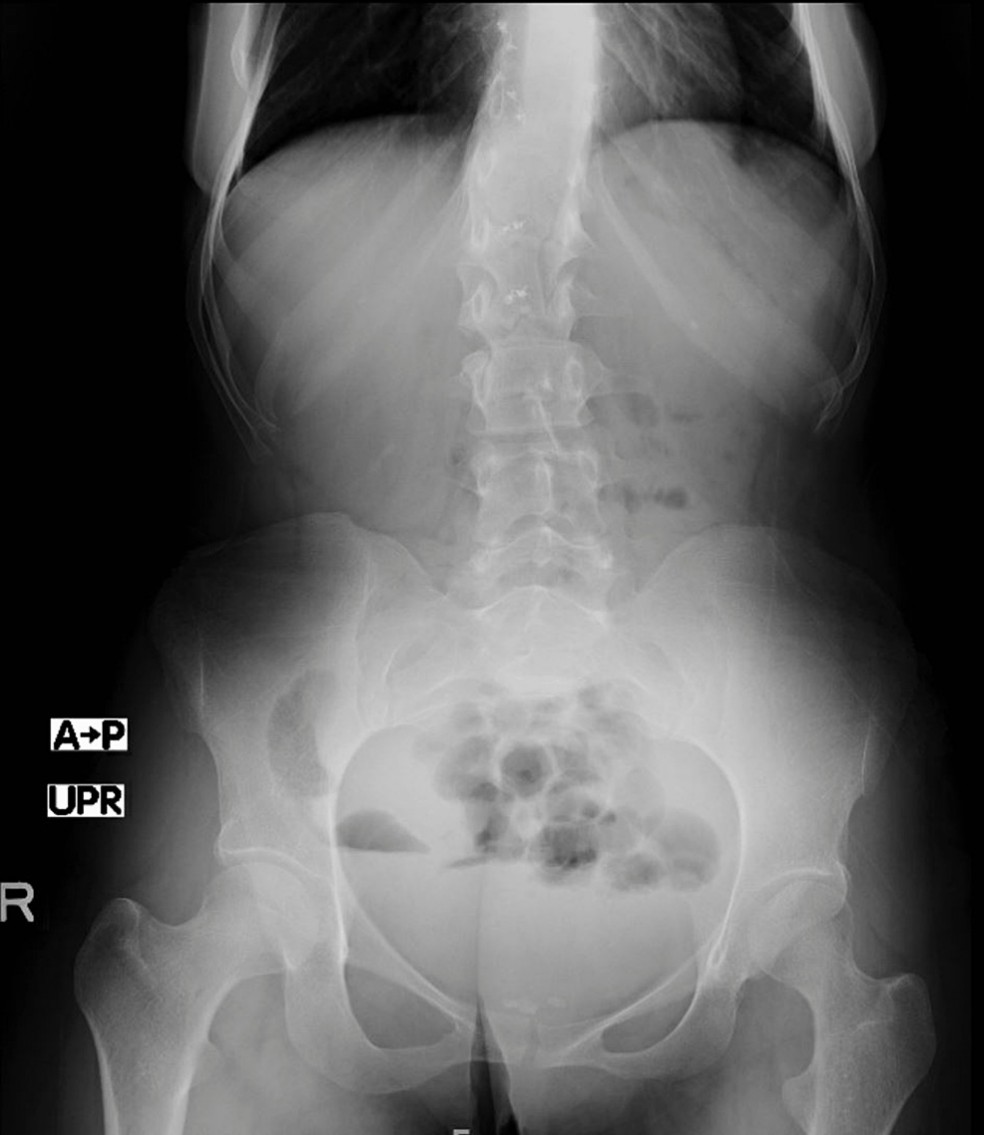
Abdominal X-ray showed no signs of air-fluid level or pneumoperitoneum
